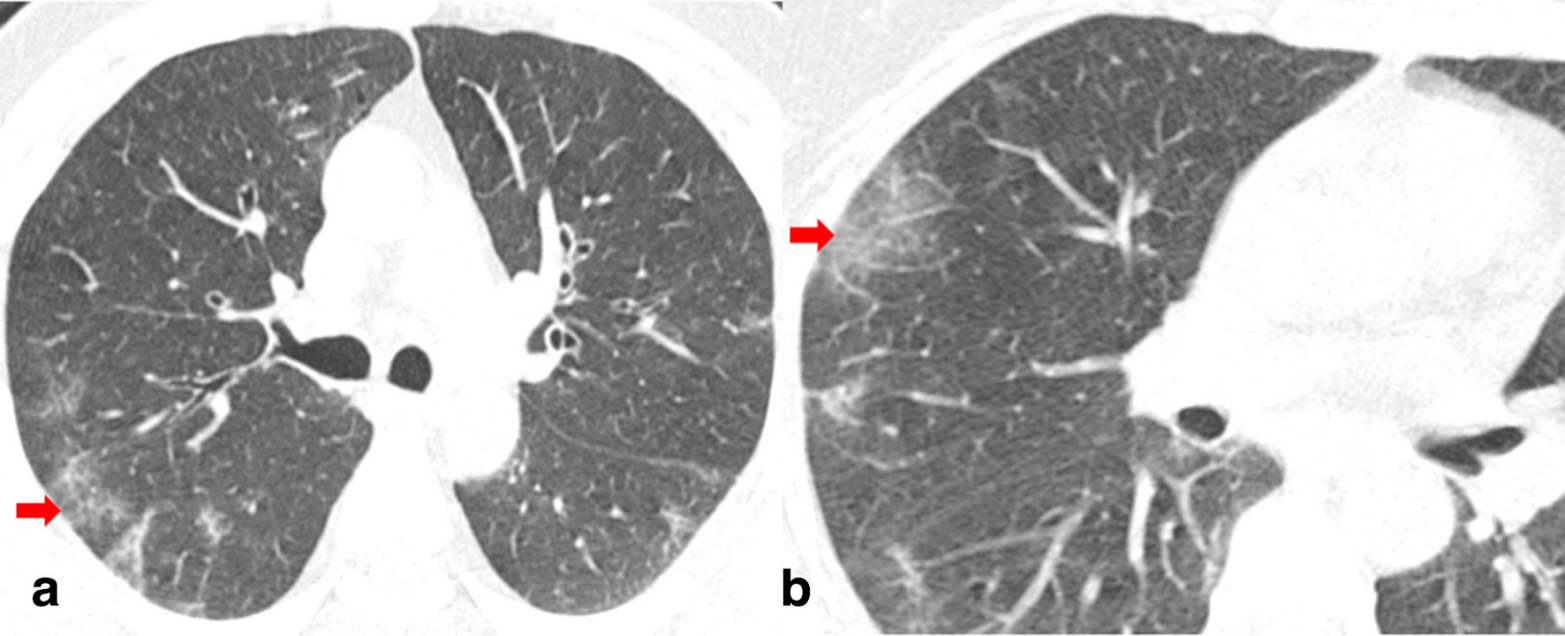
Non-contrast chest CT axial image (a) in the lung window setting of a 49-year-old male COVID-19 positive patient admitted in routine ward (clinically stable), showing few bilateral patchy ground glass opacities with fine reticulations (red arrow). Fig. b is the zoomed-in view of the same patient at a lower level showing GGO (red arrow) with fine reticulations. COVID-19, Corona virus disease 2019; GGO, ground glass opacity.
